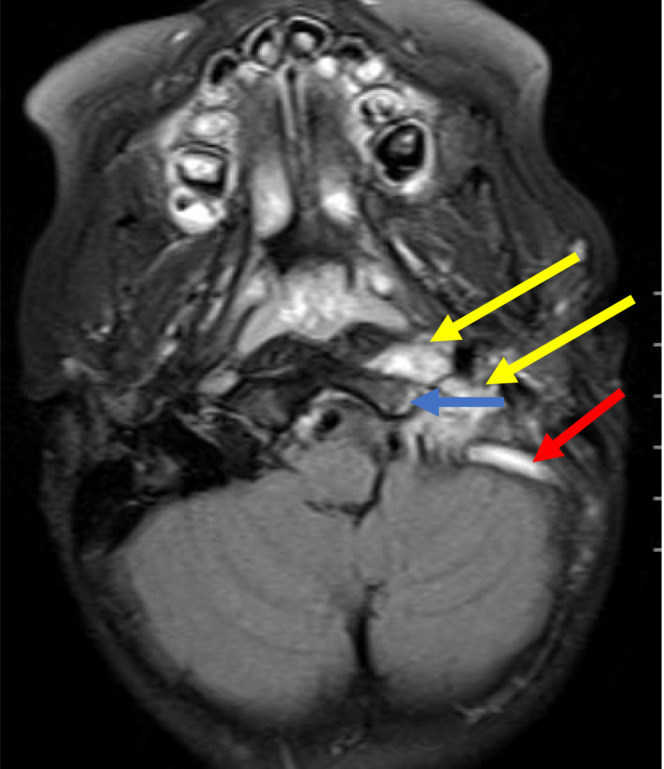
Axial T2 FLAIR image demonstrating abnormal soft tissue edema and associated retropharyngeal lymphadenopathy (yellow arrow). Associated bone marrow edema involving the left occipital bone (blue arrow). Also, note the lack of T2 flow void in the sigmoid sinus on the left–this is compatible with a thrombus (red arrow).
